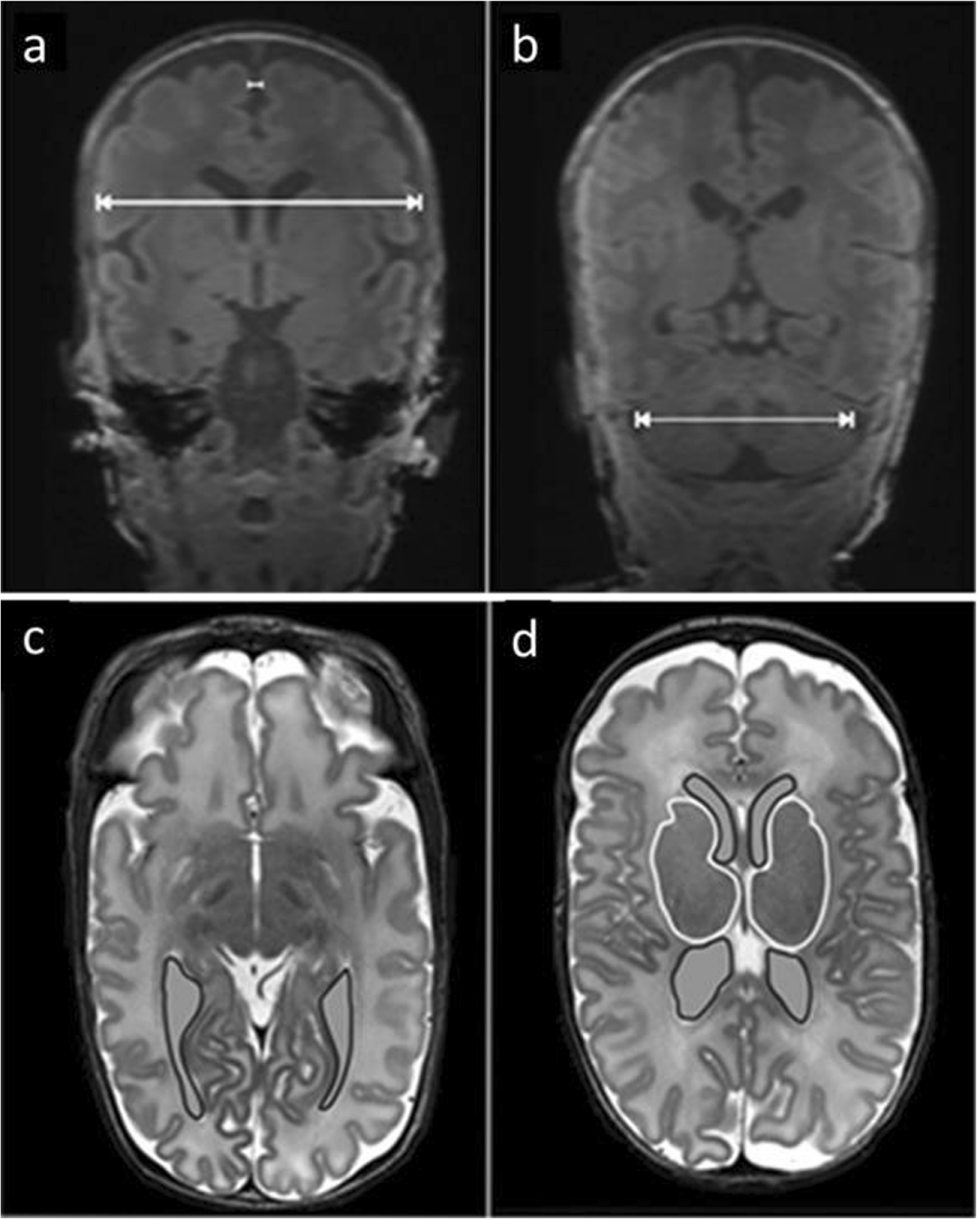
Examples of MRI measurements. a, b Measures of growth using brain metrics: interhemispheric distance (l—l; a), biparietal width (←→; a) and transcerebellar diameter (←→; b) on coronal T1-weighted images. These diameters were measured at the level of the 3rd ventricle, cochlea, and basilar artery (a) and at maximal width of the cerebellar diameter (b). c, d Volumetric measures of the lateral ventricles (black line; c, d) and the deep gray matter (white line; d) on an axial T2-weighted image, performed in every axial slice with lateral ventricles, respective deep gray matter visible
